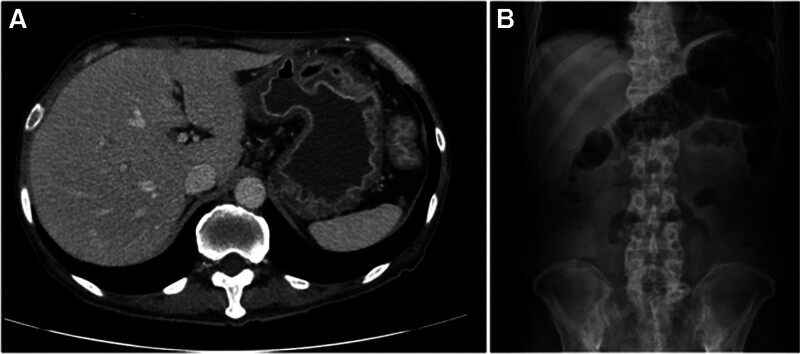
Abdominopelvic-computed tomography (AP-CT) and upright abdominal radiograph findings in a patient with gastrointestinal amyloidosis. (A) AP-CT demonstrates diffuse concentric thickening of the entire gastric wall with mucosal enhancement. (B) Upright radiograph shows multiple air-fluid levels and small bowel dilatation, suggestive of paralytic ileus.
